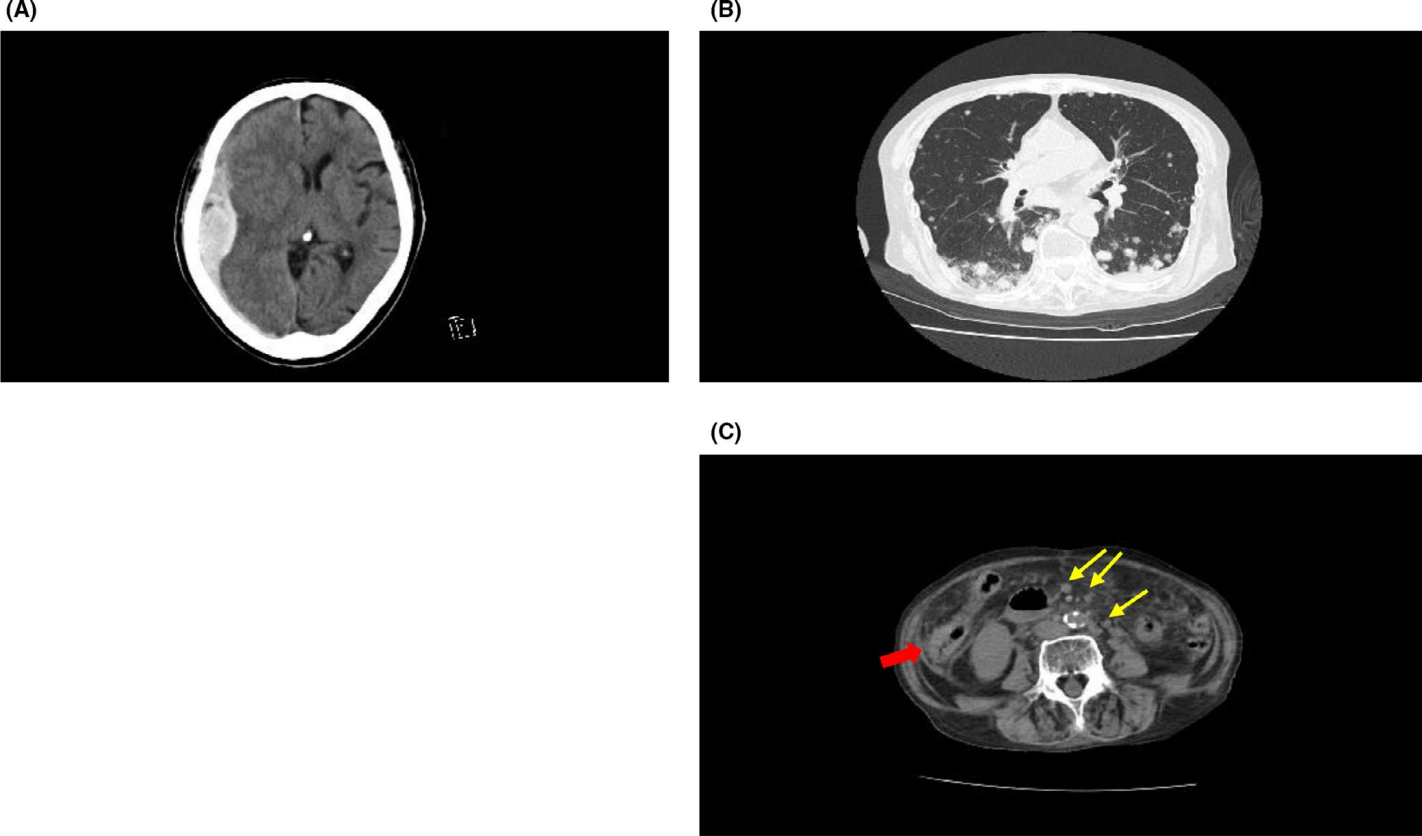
A, Computed tomography of brain in Case 1; chronic subdural hematoma. B, Computed tomography of lungs in Case 2; multiple nodular lesions in the middle to lower lobes of both lung. C, Computed tomography of abdomen in Case 2; suspected ascending colon cancer (red arrow) and para‐aortic lymphadenopathy (yellow arrows)
